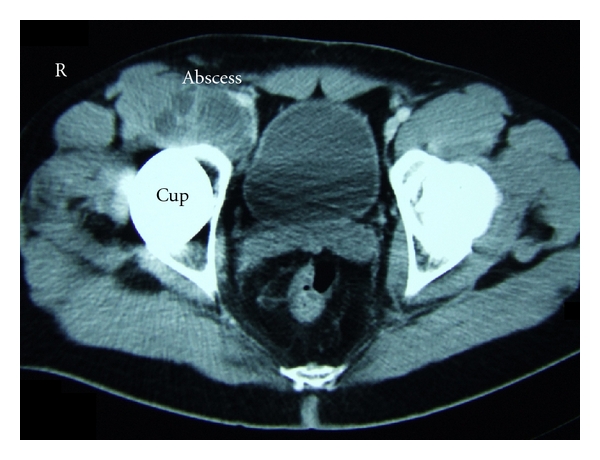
Abscess in front of the hip arthroplasty due to *S. lugdunensis.* The computer tomography (CT) shows a large cystic formation ventral to the hip reaching the psoas-muscle.
